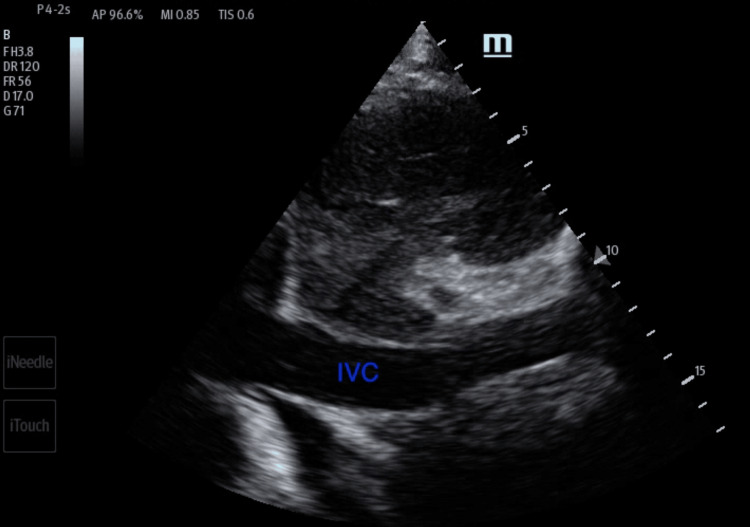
Distended inferior vena cava visualized on transthoracic echocardiogram. IVC: inferior vena cava
